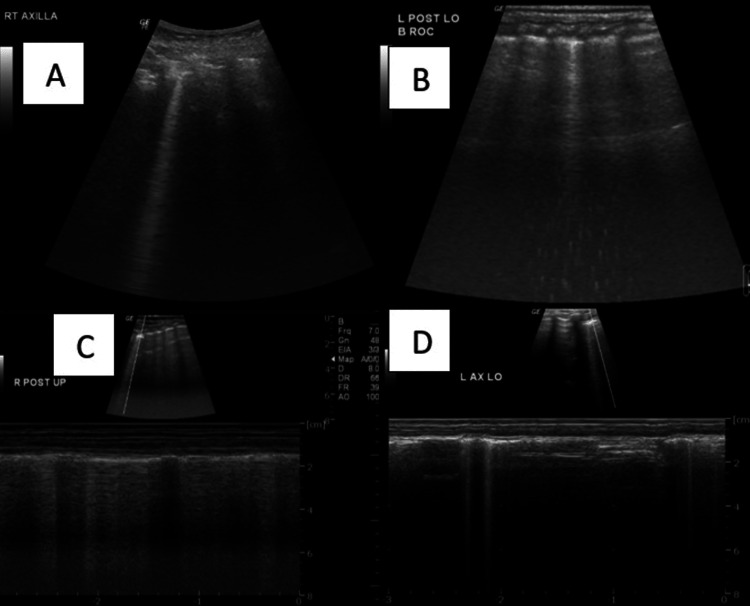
A) B-mode ultrasound in the right axillary region shows a single B line; B) B-mode ultrasound in the left posterior lower region shows a single B line; C,D) M-mode ultrasound confirming the presence of the B line. B mode: Brightness mode; M mode: Motion mode
